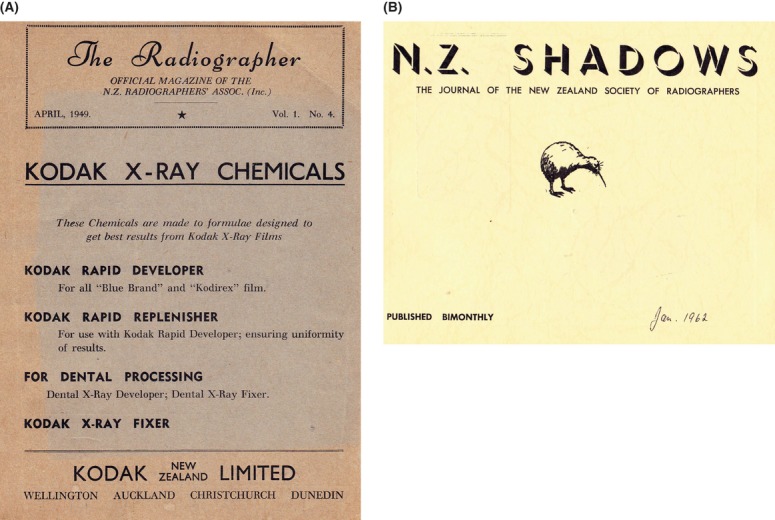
New Zealand: (A) 1949 cover page of *The Radiographer* and (B) 1962 cover page of *Shadows*.
